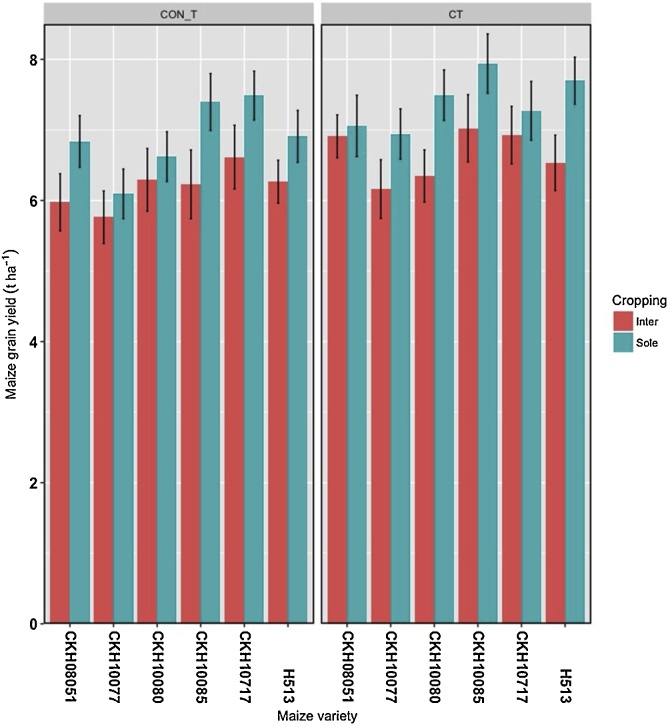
Productivity of six genotypes in sole and intercropping with cowpea and under conventional (CT) and conservation tillage (CON_T) practices for six cropping seasons (2013–2016) in on-station trials at Kiboko, Kenya.
